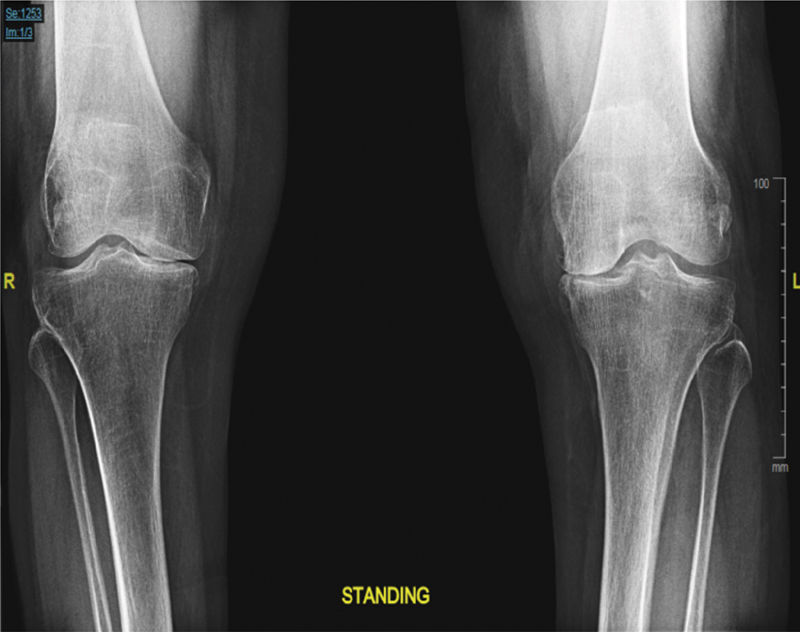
Standing anteroposterior X-ray of both knees, showing severe bilateral tricompartmental osteoarthritis.
